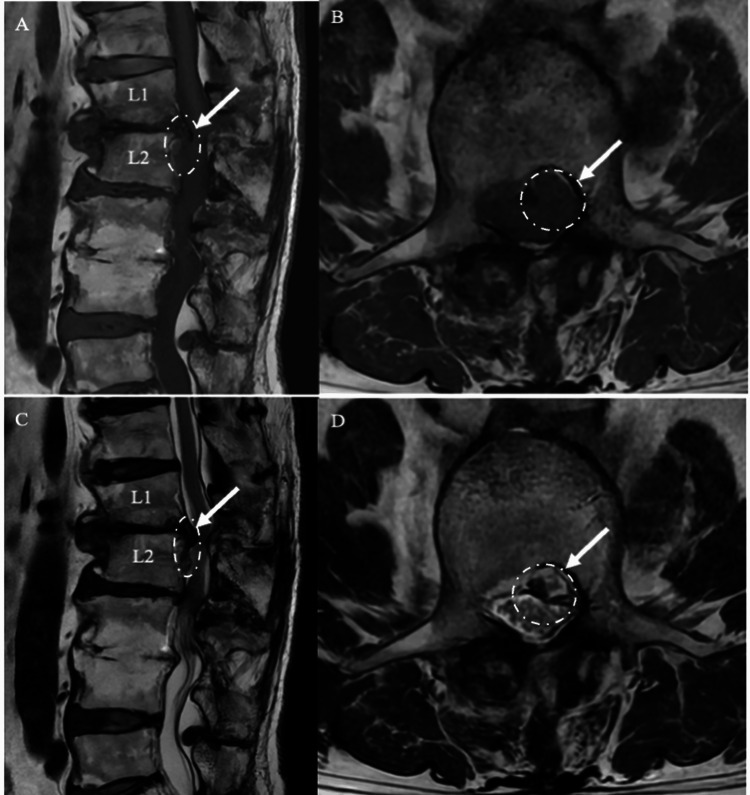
Postoperative MRI at One-Year Follow-up Sagittal and axial T1-weighted (A, B) and T2-weighted (C, D) MRI scans obtained one year after surgery demonstrate complete resolution of the epidural gas at the surgical site with no signs of recurrence *(white arrow *and *dotted circle)*.
